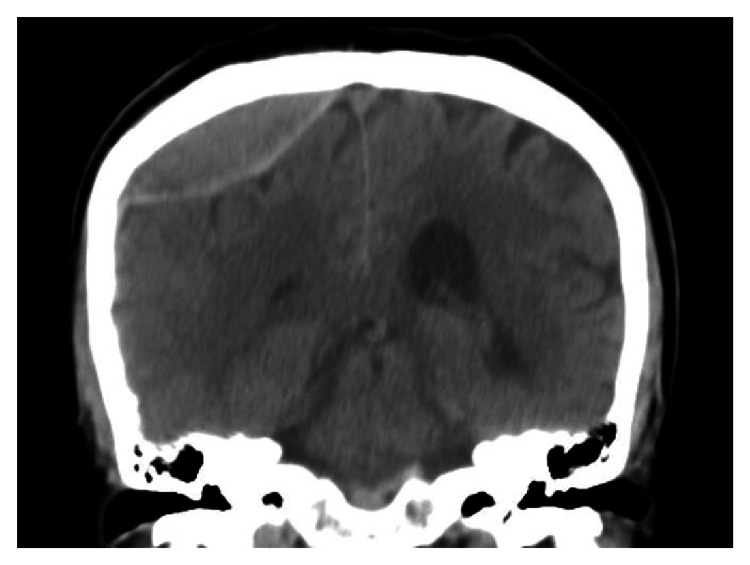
An epidural hematoma on a coronal CT image from a different patient. The image shows its biconvex shape and approximate bilateral symmetry.
